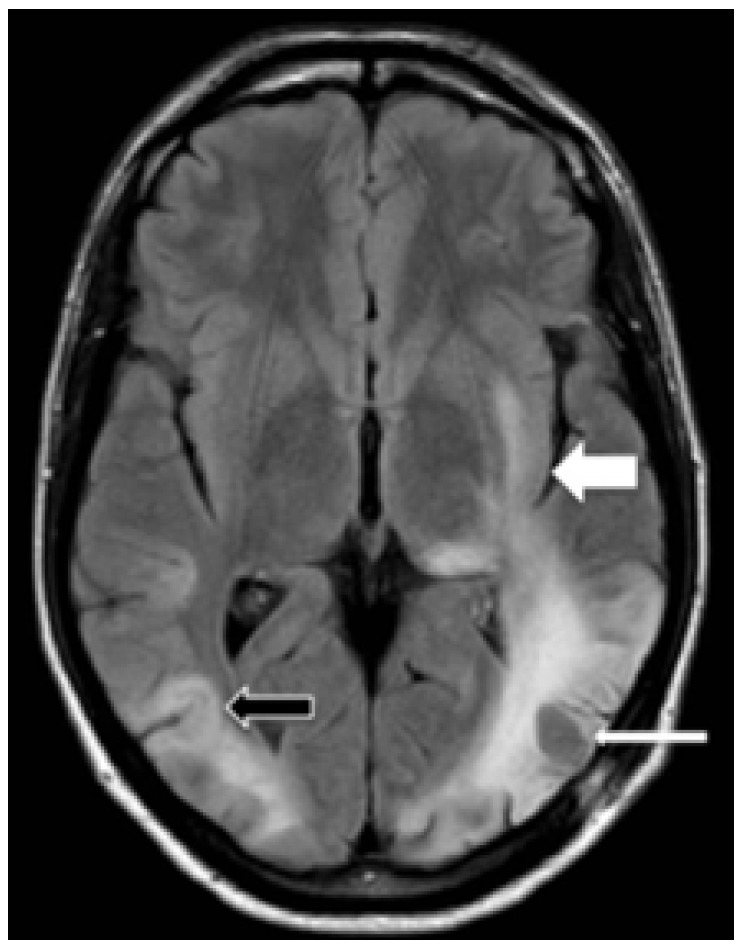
FLAIR image reveals multifocal and asymmetric progression of PML, with involvement of the left temporal-occipital lobes (white arrow) and of the subcortical region of the right temporal lobe (U-fibers) (black arrow). Previous biopsy area (thin white arrow).
